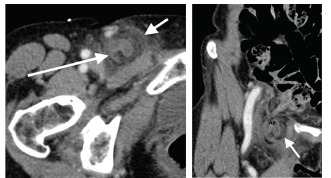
(Left) Axial view of the inflamed appendix within the hernia sac on computed tomography. Long arrow pointing to the appendix, short arrow pointing to the hernia sac. (Right) Coronal view of appendicitis within the hernia sac on computed tomography (arrow).
